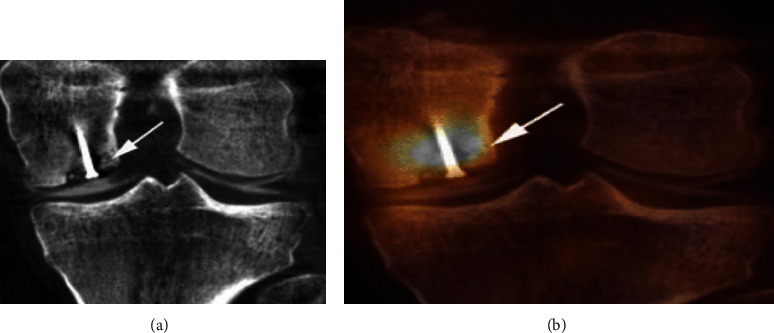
(a) The SPECT/CT scan of the knee joint shows clear articular cartilage. (b) The SPECT/CT arthrography image of the knee joint showing enhance the value of SPECT/CT screening.
